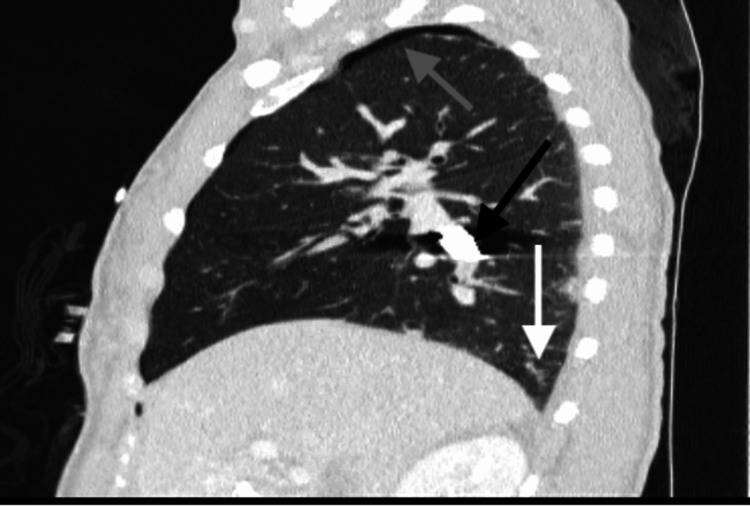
Sagittal CT chest reveals bullet is within the right lower lobar pulmonary artery (black arrow). Small right hemopneumothorax is partially visualized (gray arrow and white arrow).
